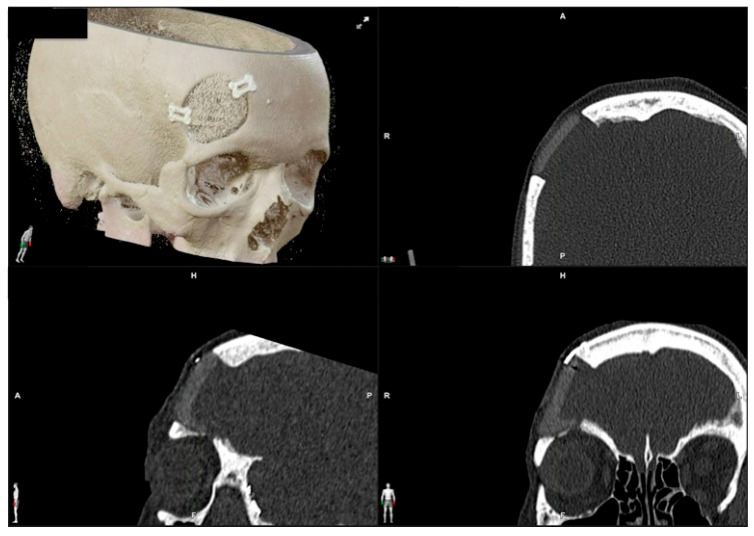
Postoperative CT scan. Three-dimensional, axial, sagittal and coronal views can be appreciated.
